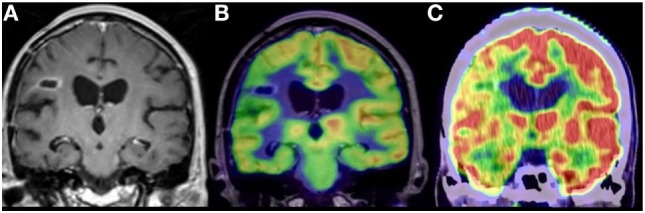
Anaplastic astrocytoma, status post surgery, radiation, and chemotherapy. (A) Contrast-enhanced coronal MR image demonstrating nodular enhancement about a right frontal operculum cavity. (B) Simultaneous co-registered positron emission tomography (PET)-MR image accurately demonstrating that the area of nodular enhancement has no associated elevated fluoro-2-deoxy-d-glucose (FDG) avidity. (C) Coronal image from a fused FDG PET-CT demonstrating no elevated FDG avidity in the region of nodular enhancement, although the anatomical resolution is limited compared to the PET-MR. No copyright permissions were required for use of these images.
